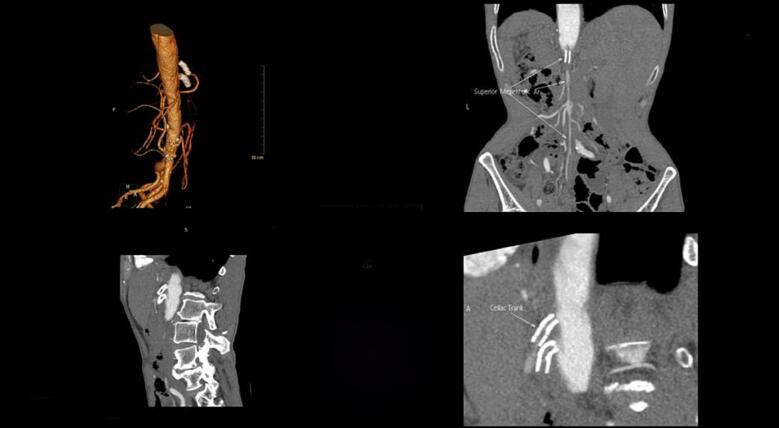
Abdominal CT angiography of patient before the surgery showing absence of blood flow in the stented SMA and celiac trunk and also run off of SMA.
